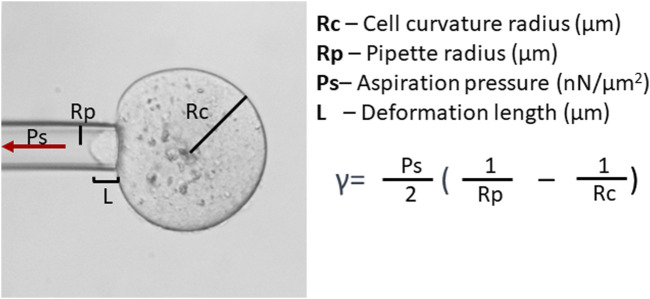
Cortical tension (CT, γ) analysis. CT analysis can be conducted by micropipette aspiration. Assessment of CT requires the measurement of cell curvature radius and aspiration pressure (Ps) when the deformation length (L) becomes equal to the micropipette radius (Rp) and utilizes the Young–Laplace equation.
